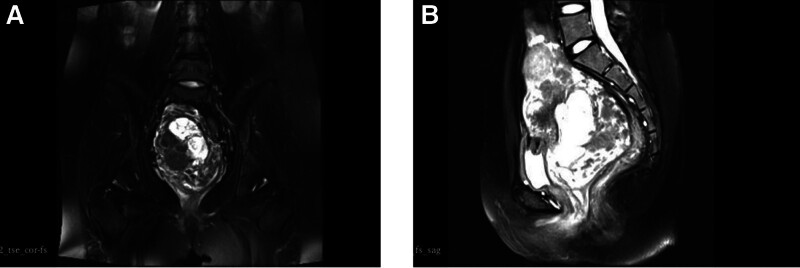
MRI images of patient 2. MRI = magnetic resonance imaging.
